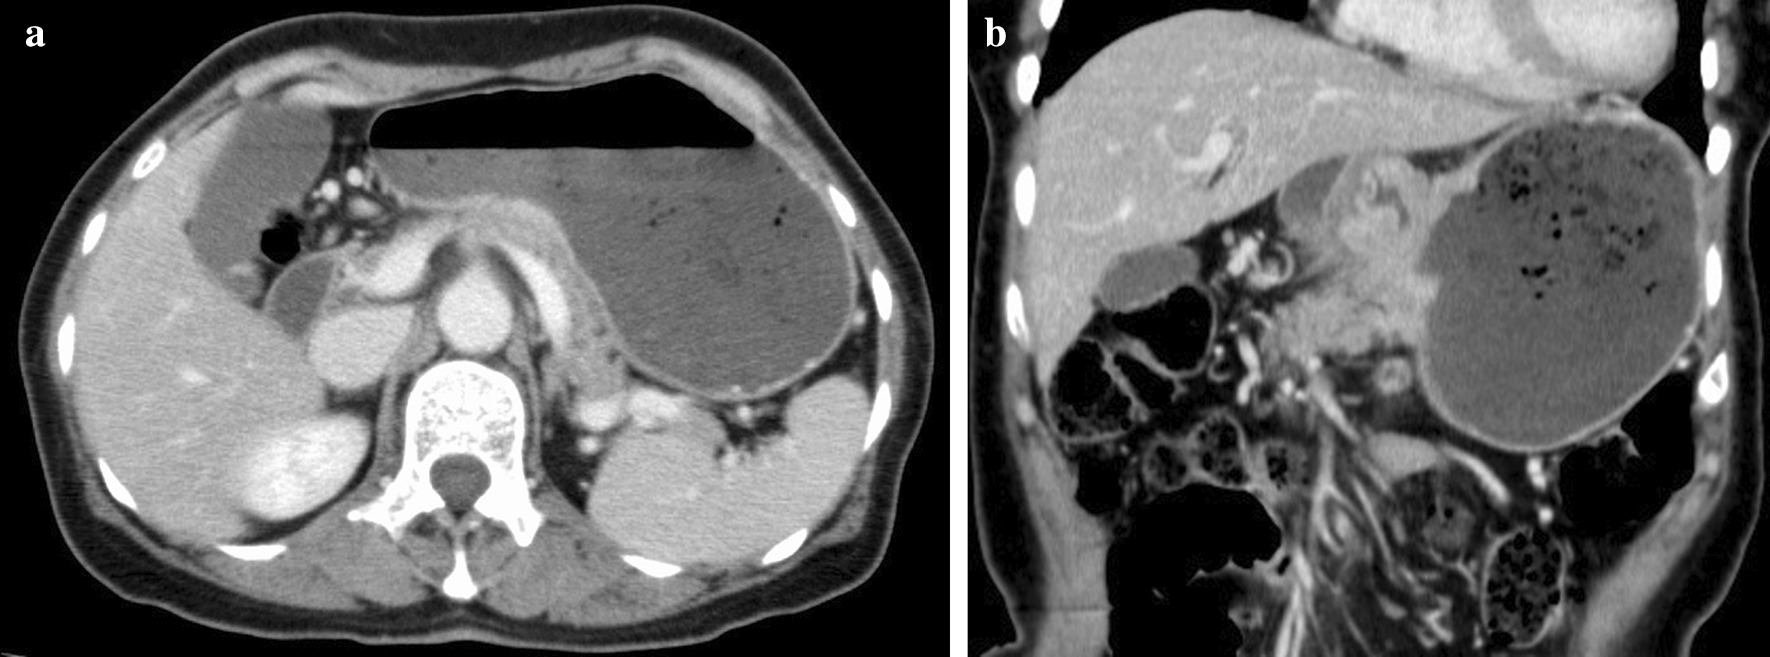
a Computed tomography (coronal plane). Suspected infiltration of the tumor into the pancreas. b Computed tomography (axial view of the same location as in Fig. 1-1)
